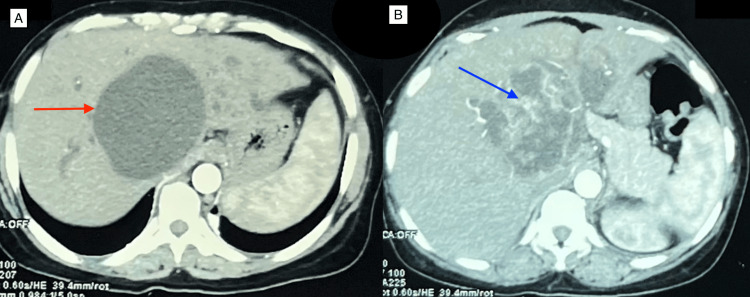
CECT abdomen (A) Image showing a large cystic lesion (red arrow) in segments 2, 3 and 4 extending to segments 5 and 8 of the liver. (B) The solid components show a significant contrast enhancement (blue arrow). CECT: Contrast-enhancement computed tomography
